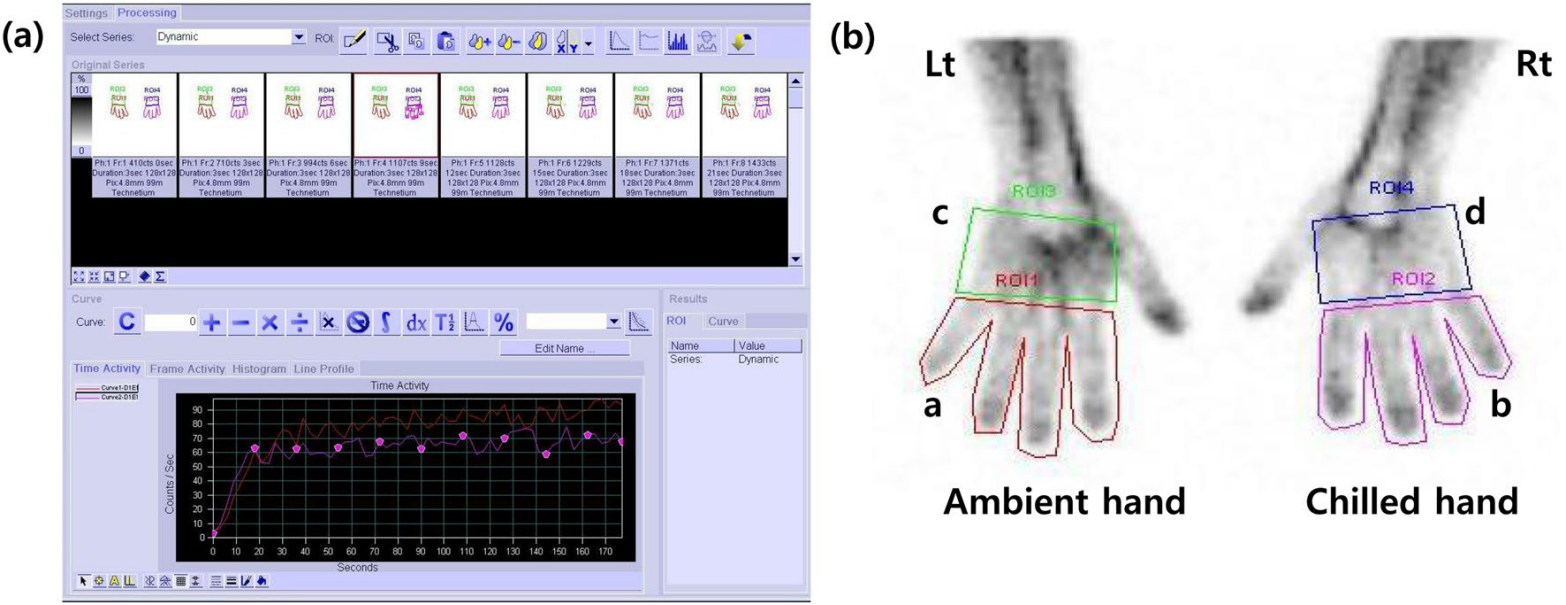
(a) ^99m^Tc-HDP blood flow scintigraphy in a patient with Raynaud’s phenomenon. (b) An example of regions of interest (ROI) for calculating chilled–ambient uptake ratios. Four ROIs were manually drawn around all fingers except the thumb (a,b) and palmar area (c,d) of both hands.
